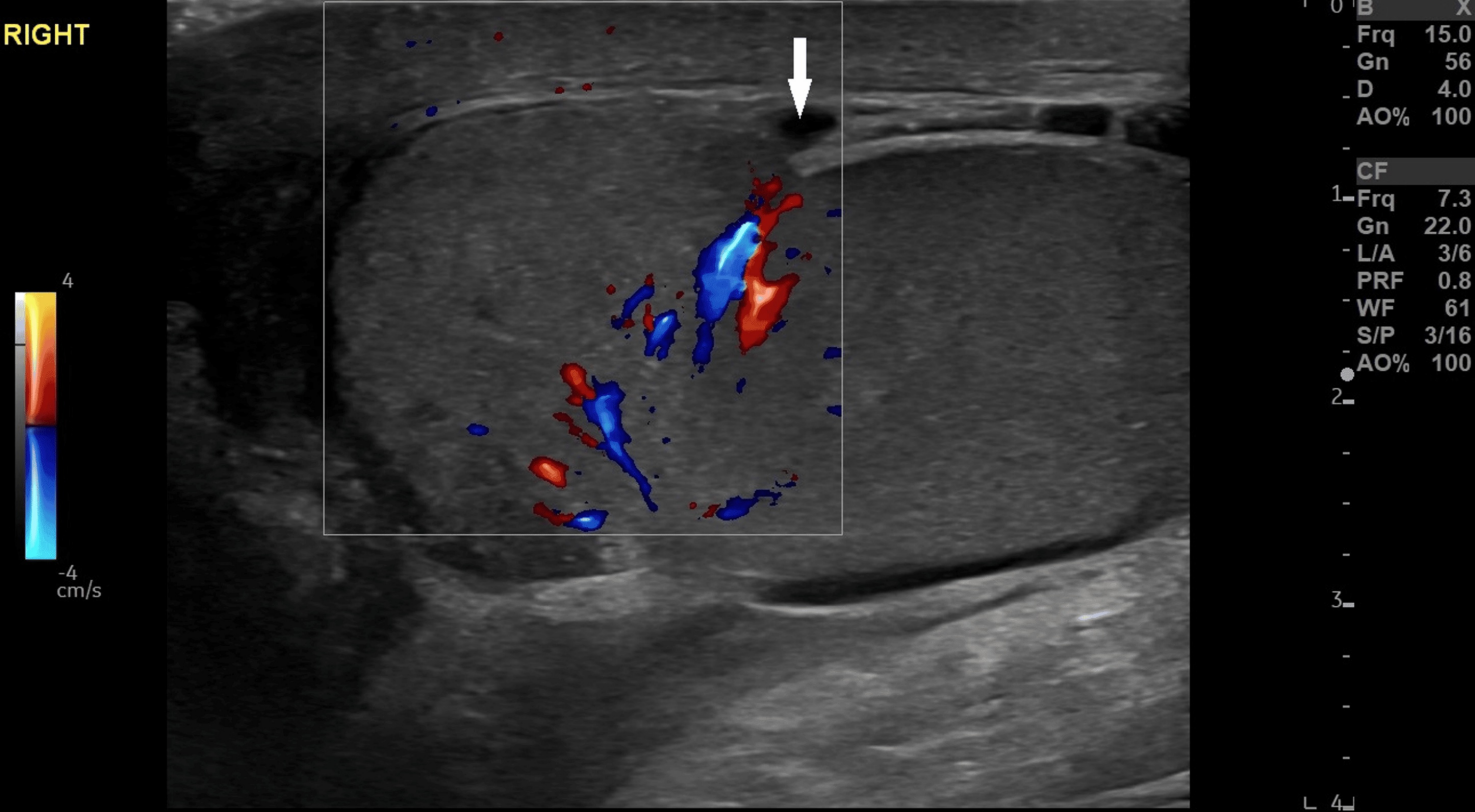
Testicular rupture. Gray-scale and color Doppler image of the right testis exhibited focal discontinuity of the tunica albuginea (arrow) in association with heterogeneous testicular echotexture.
